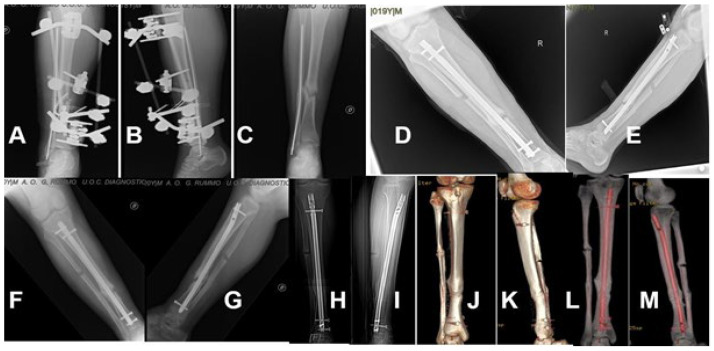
(A,B) 21-year-old man fallen from motorcycle, open GA IIIB tibia fracture, treated with Modular External Fixator in emergency. (C) Aseptic nonunion after 7 months of tibia. (D,E) Postoperative X-rays, after the reamed nailing and high fibular osteotomy. (F,G) One month after the surgery the bone callus on 3rd cortex appeared on X-rays. (H,I) At 3 months, the X-rays showed a good bone callus at the moment of nailing dynamization. (J–M) At 6 months, 3D CT scan showed the perfect formation of RUST.
